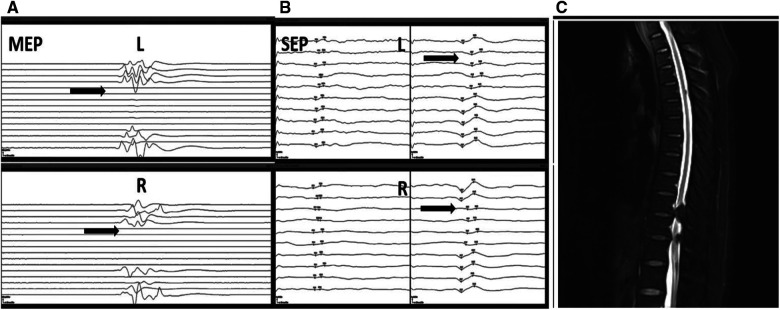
A typical sample case from a 59-year-old adult woman with T10-T12 TOLF with IONM alert and recovery. During the operation, the patient experienced rapid loss of MEP in both lower extremities (A). At the same time, the SSEP amplitude decreased by more than 50%, and the latency was prolonged by more than 10% (B). A dual-mode alarm signal appeared, and then IONM recovered. The patient's preoperative MRI images showed TOLF of the patient at T10-T12 with severe spinal cord compression (C). TOLF, thoracic ossification of ligamentum flavum; IONM, intraoperative neurophysiologic monitoring; MEP, motor-evoked potential; SSEP, somatosensory-evoked potential; MRI, magnetic resonance imaging.
